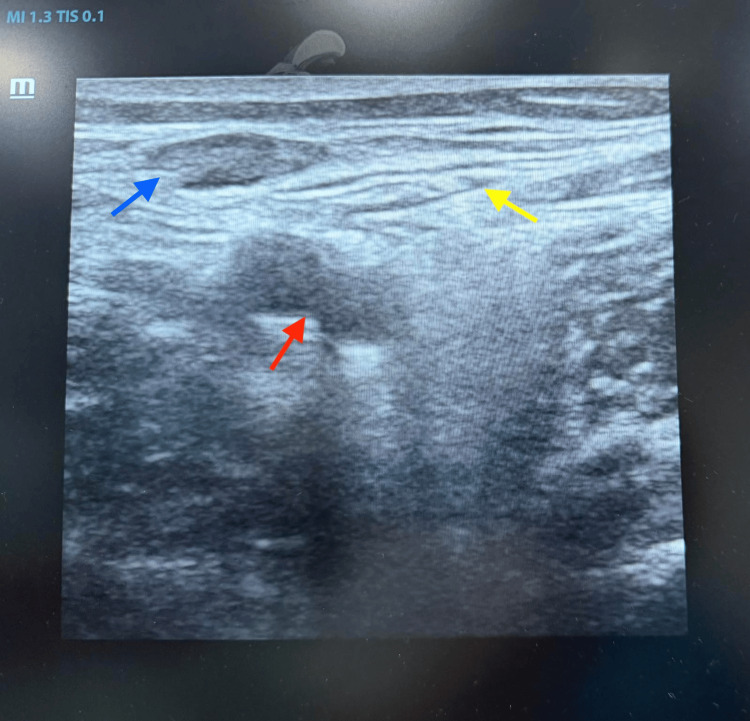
Ultrasound image of the femoral neurovascular bundle. Ultrasound image demonstrating the femoral neurovascular structures. The femoral artery (red arrow) is seen as a pulsatile anechoic structure, the femoral vein (blue arrow) lies medial to the artery, and the femoral nerve (yellow arrow) appears as a hyperechoic structure lateral to the artery.
